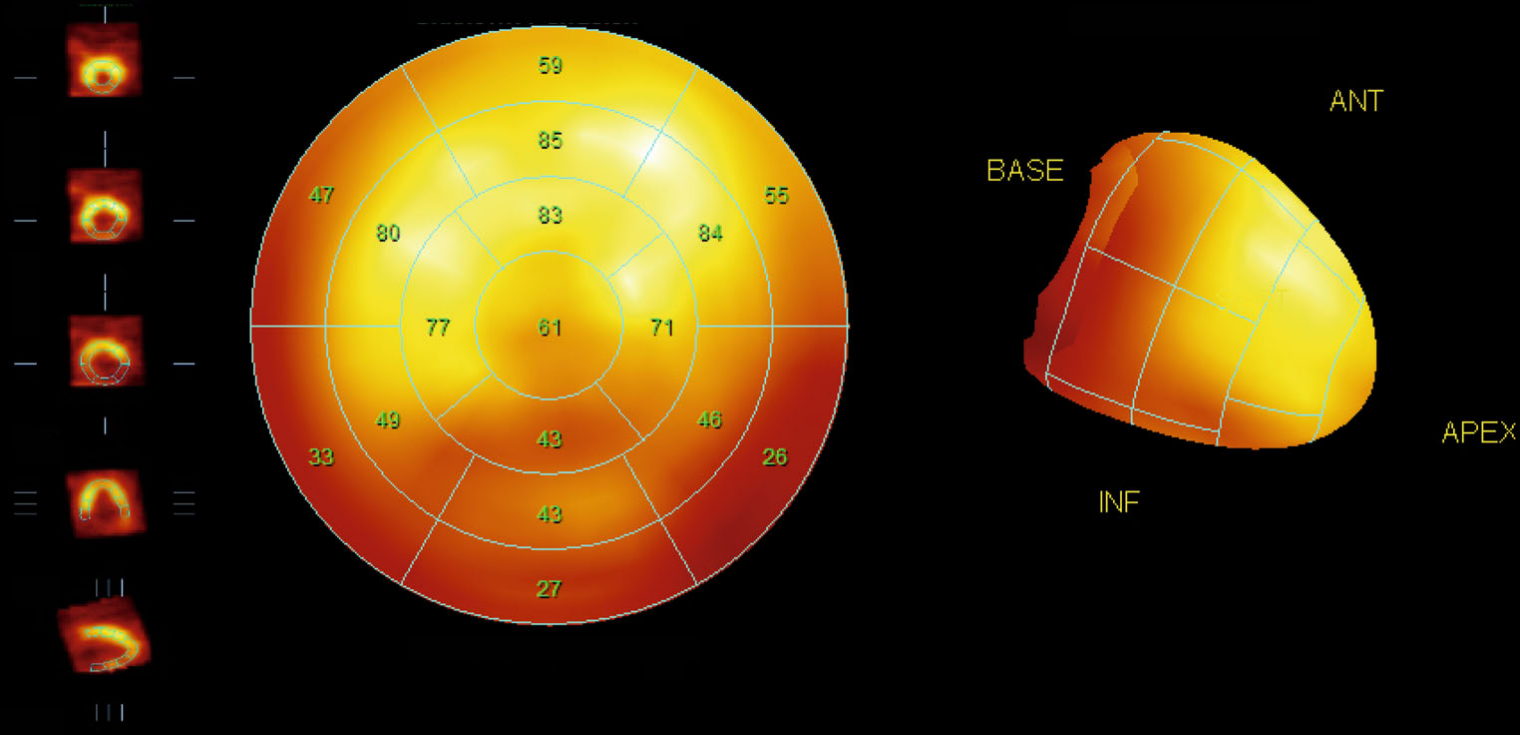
Example of late ^123^I-*m*IBG SPECT imaging. On the left the conventional short, vertical and horizontal axis, in the middle the corresponding 17-segment model polar map and on the right a 3D reconstruction. There is impaired regional ^123^I-*m*IBG uptake in the inferior wall from the myocardial base until the apex with extension to both inferoseptal and inferolateral regions
